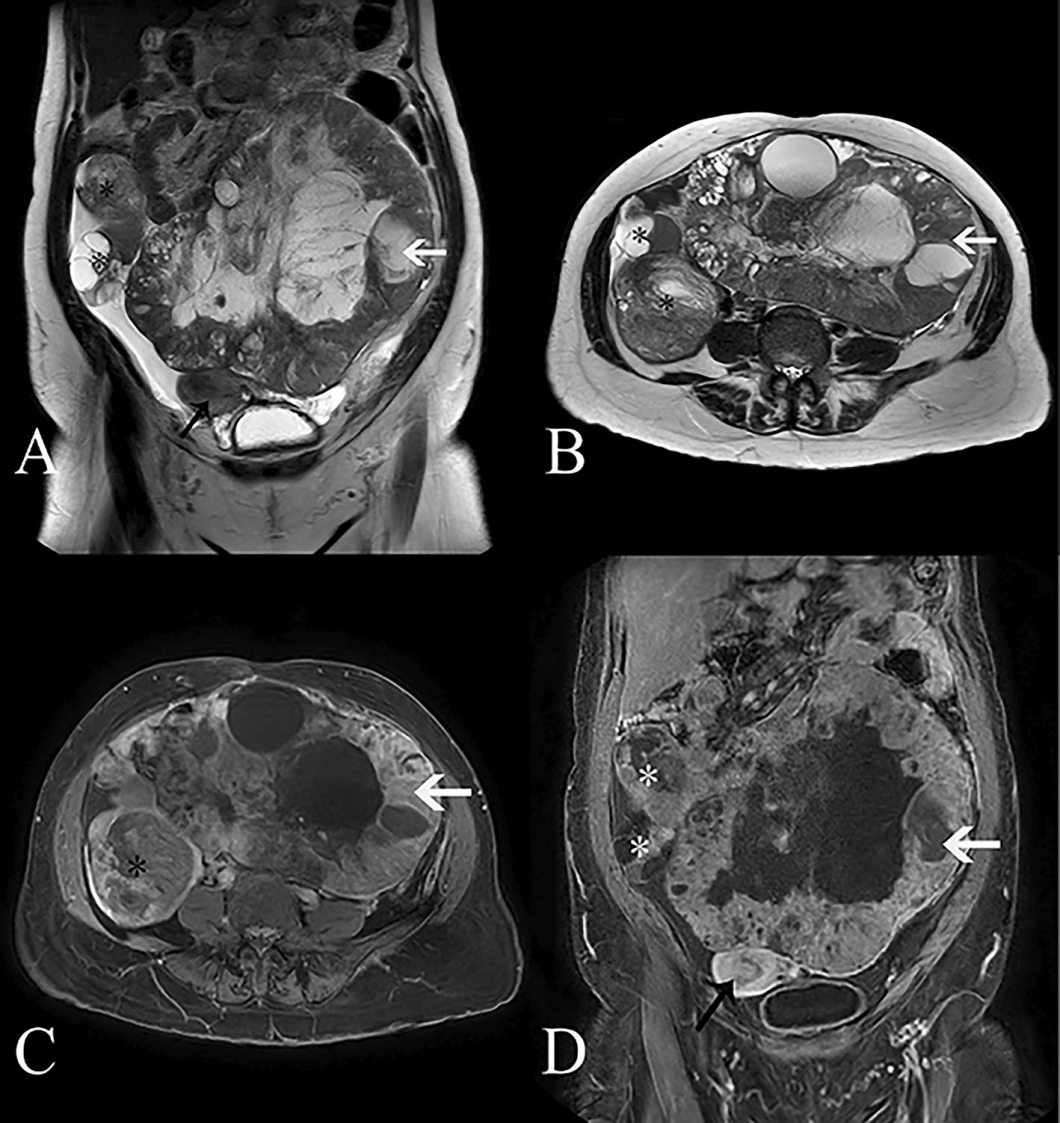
Image of MRI (A) on the coronal T2‐weighted sequence, the left ovarian tumor (indicated by the white arrow) exhibits cystic and solid changes with mixed high and low T2 signals. Similarly, the right ovarian tumor (indicated by *) also displays cystic and solid changes with mixed high and low T2 signals. The uterus (indicated by the black arrow) is compressed and shifted toward the lower right. (B) Axial T2‐weighted sequence shows cystic and solid changes in the left ovarian tumor (indicated by the white arrow), with mixed high and low T2 signals. Similarly, the right ovarian tumor (*) also exhibits cystic and solid changes with mixed high and low T2 signals. (C) Coronal T1‐weighted enhanced sequence demonstrates significant and inhomogeneous enhancement in the solid portion of the left ovarian tumor (indicated by the white arrow), while the cystic portion shows no significant enhancement. The solid part of the right ovarian tumor (*) also exhibits significant and inhomogeneous enhancement, with no apparent enhancement in the cystic portion. (D) Axial T1‐weighted enhanced sequence reveals significant and inhomogeneous enhancement in the solid part of the left ovarian tumor (indicated by the white arrow), while the cystic part does not show significant enhancement. Similarly, the solid portion of the right ovarian tumor (*) demonstrates significant and inhomogeneous enhancement, with no observable enhancement in the cystic part. The uterine muscle layer enhances uniformly.
